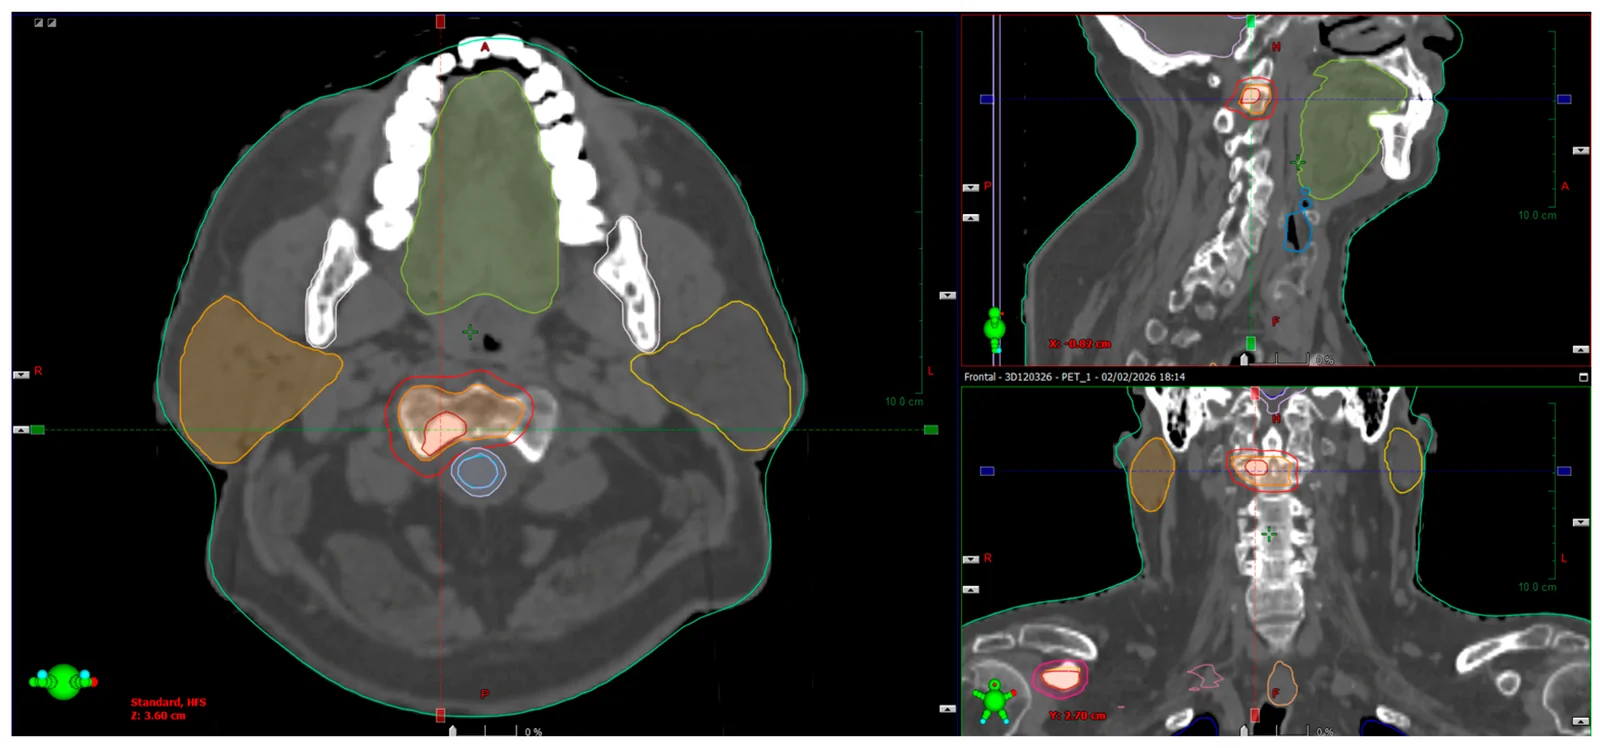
Treatment planning of SBRT directed to a PSMA-positive lesion in the second cervical vertebra (C2), administered in combination with ADT and ARSI.
